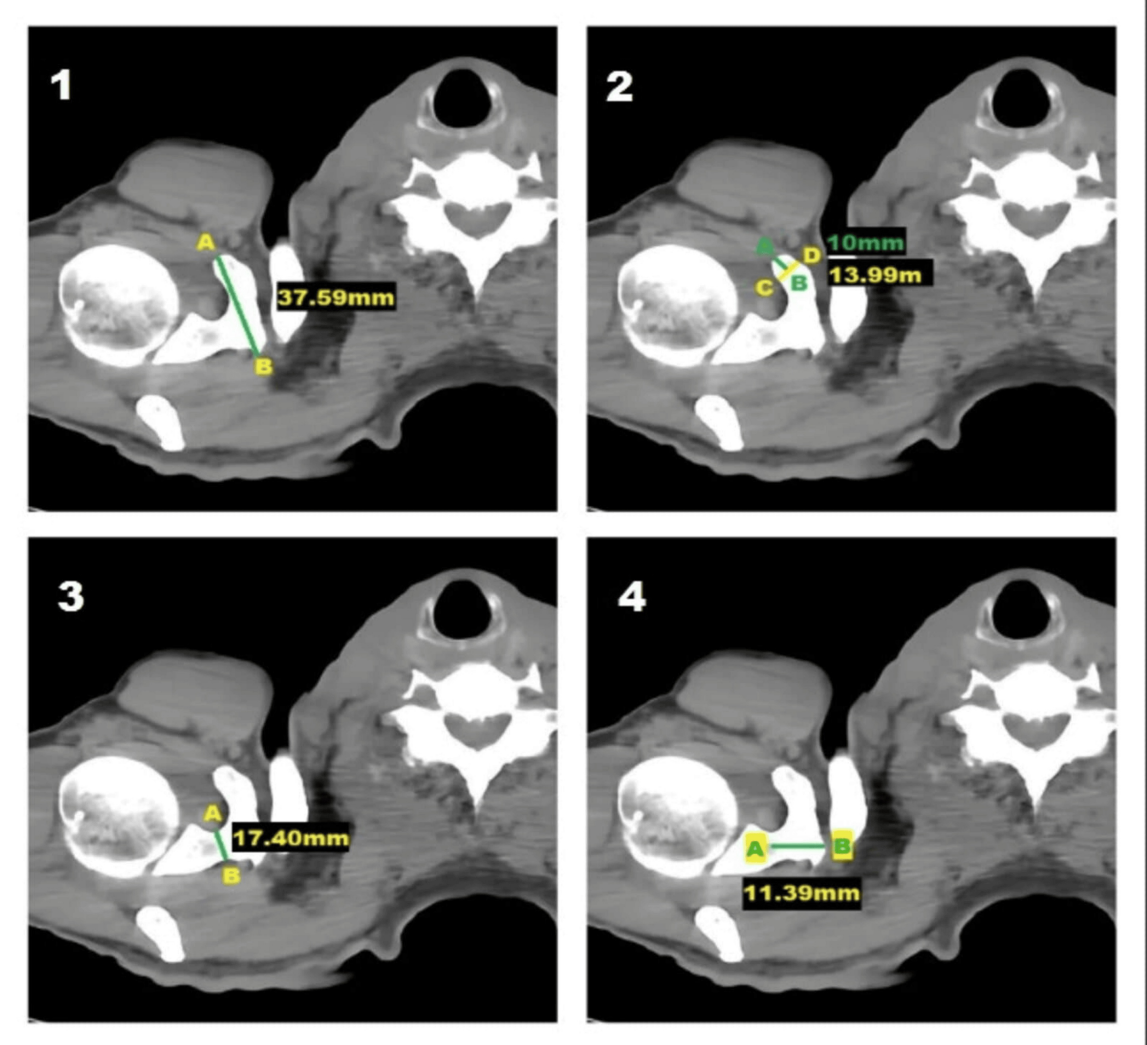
Axial CT scan image showing the measurements of the right coracoid process: (1) length, (2) tip thickness, (3) base height, and (4) base width CT axial section at the level of T1 CT: computed tomography
